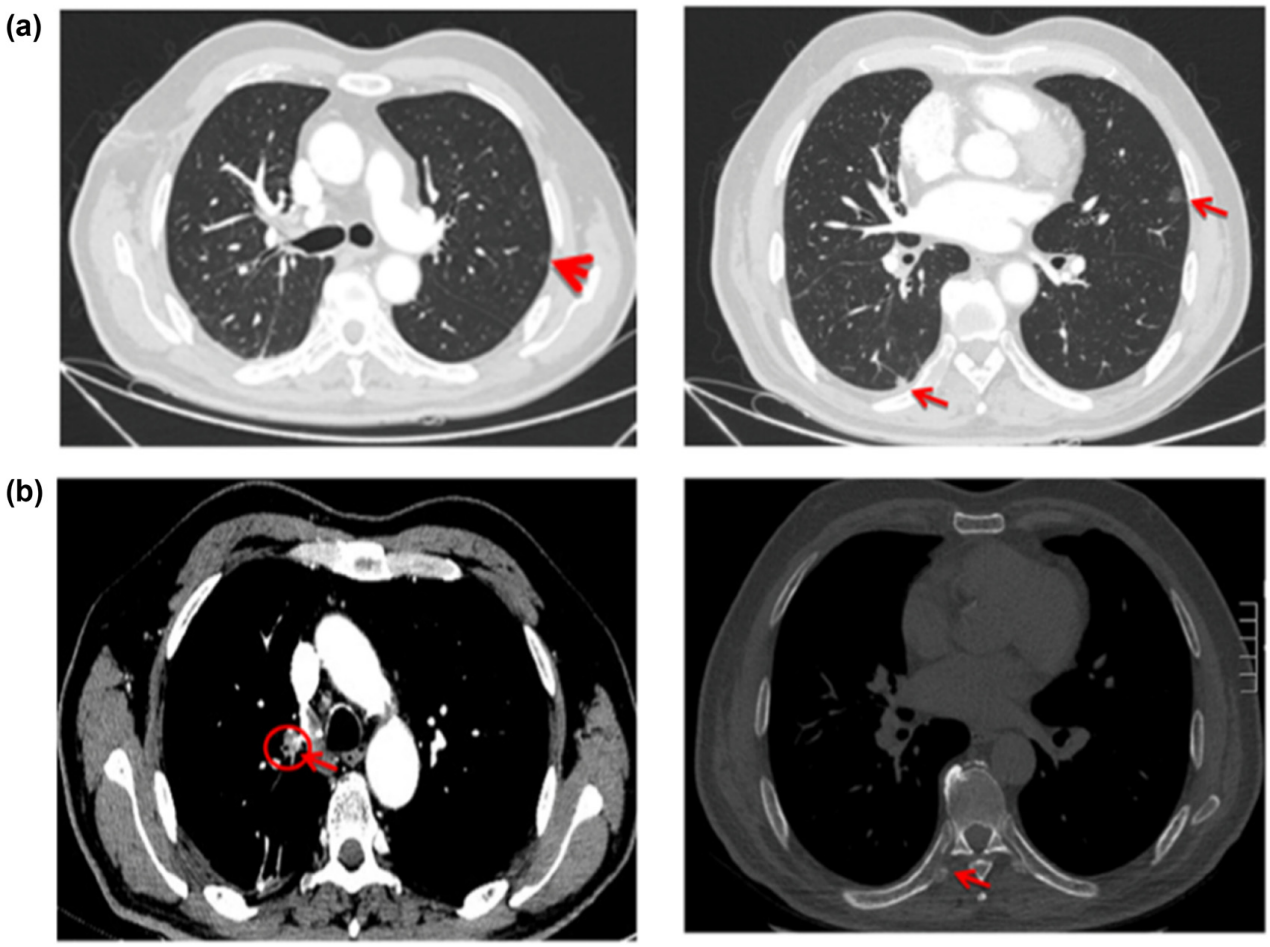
(a and b) Baseline CT of the pretreatment primary tumor lesions (July 17, 2017).
